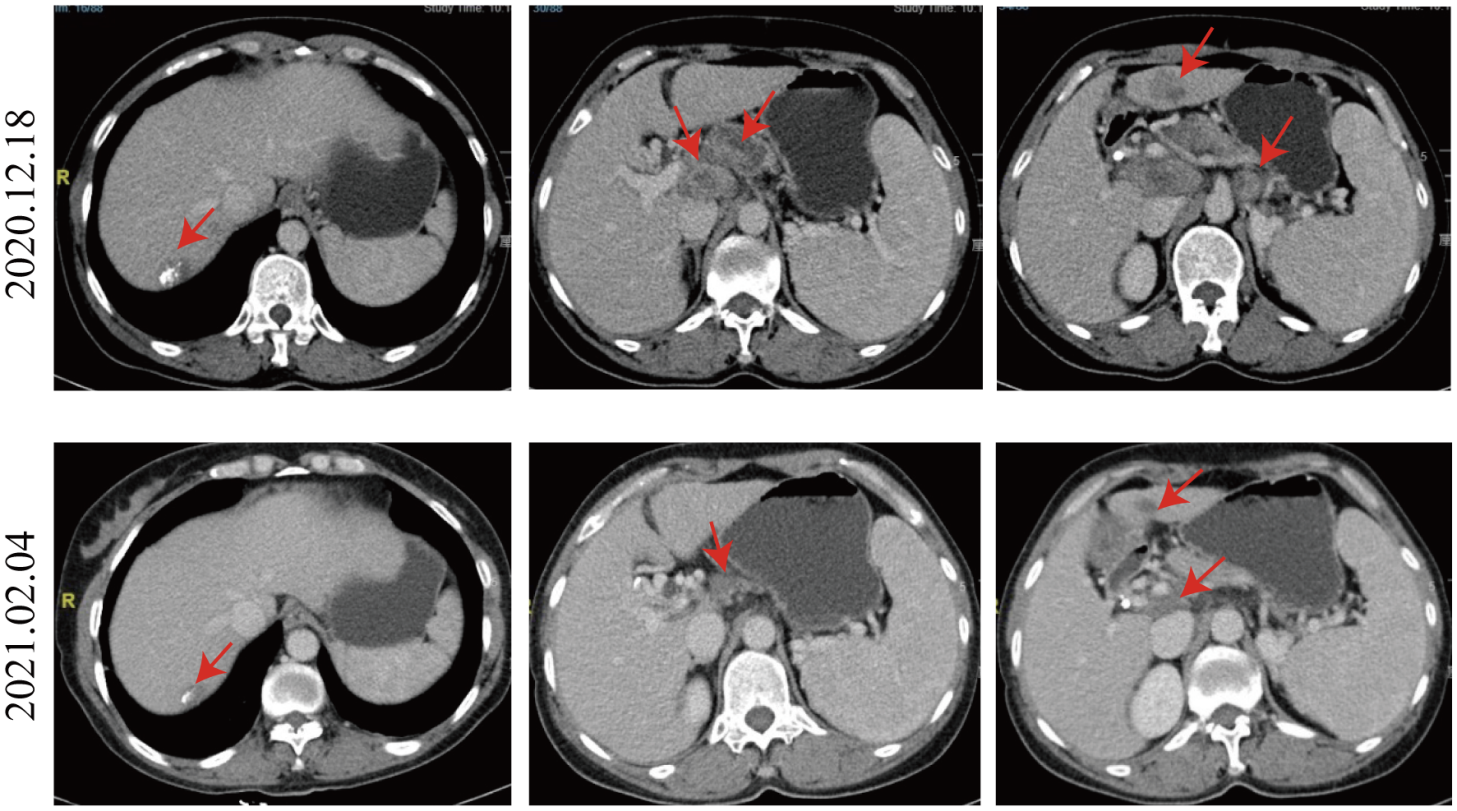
Comparison of CT images taken on December 18, 2020, and February 4, 2021. Compared to the CT image of 2020.12.18, the nodular masses of the pancreas’s neck, the liver’s hilar region, and the retroperitoneum were smaller. Multiple nodules were still present in the liver, and some exhibited slight changes following treatment, not significantly different from before.
